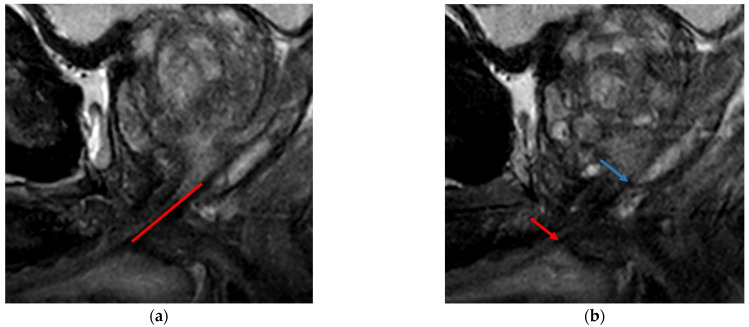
Membranous urethral length (MUL) measurement. (a) Midsagittal T2 weighted MRI image (b) Parasagittal T2 weighted MRI image. The measurement line of the MUL is shown with the red line. Regularly the lower border of the prostate (blue arrow) and upper border of the penile base (red arrow) can be better appreciated on the parasagittal images. In our center, we measure the MUL dorsal of (and parallel to) the hyperintense urethra lumen. Although this patient has a relatively long MUL, he used one pad a day after 12 months, with a score of 6 on 21 point scale for incontinence (0 = continent, 21 = very incontinent).
